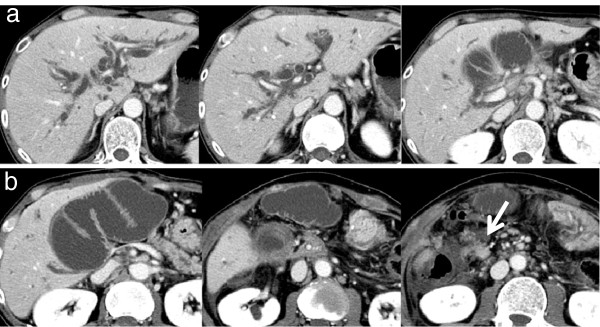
Abdominal contrast-enhanced computed tomography on admission. Abdominal contrast-enhanced computed tomography showed afferent loop obstruction and intrahepatic bile duct dilatation due to nodal recurrence (white arrow).
